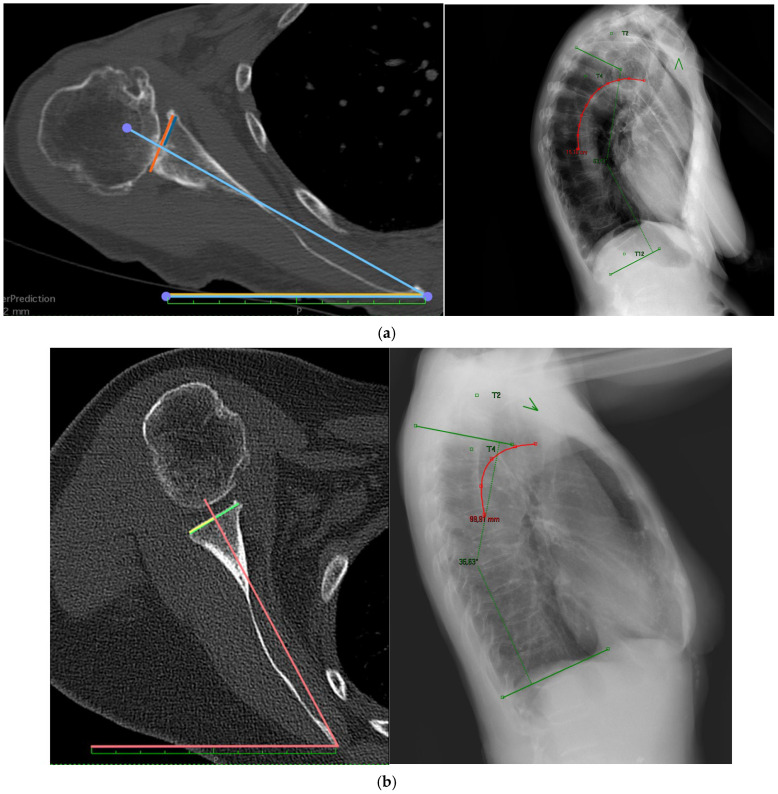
Examples of scapular inclination angle (Scapular Inclination Rotation, SIR) measurement in two patients with varying degrees of scapular tilt. (a) The measured SIR is 29.3°, and the corresponding Cobb angle is 53.43° (Type C). (b) The SIR is 51.7°, and the Cobb angle is 35.83° (Type A). Axial and sagittal CT reconstructions were used to identify anatomical landmarks and compute angular values.
